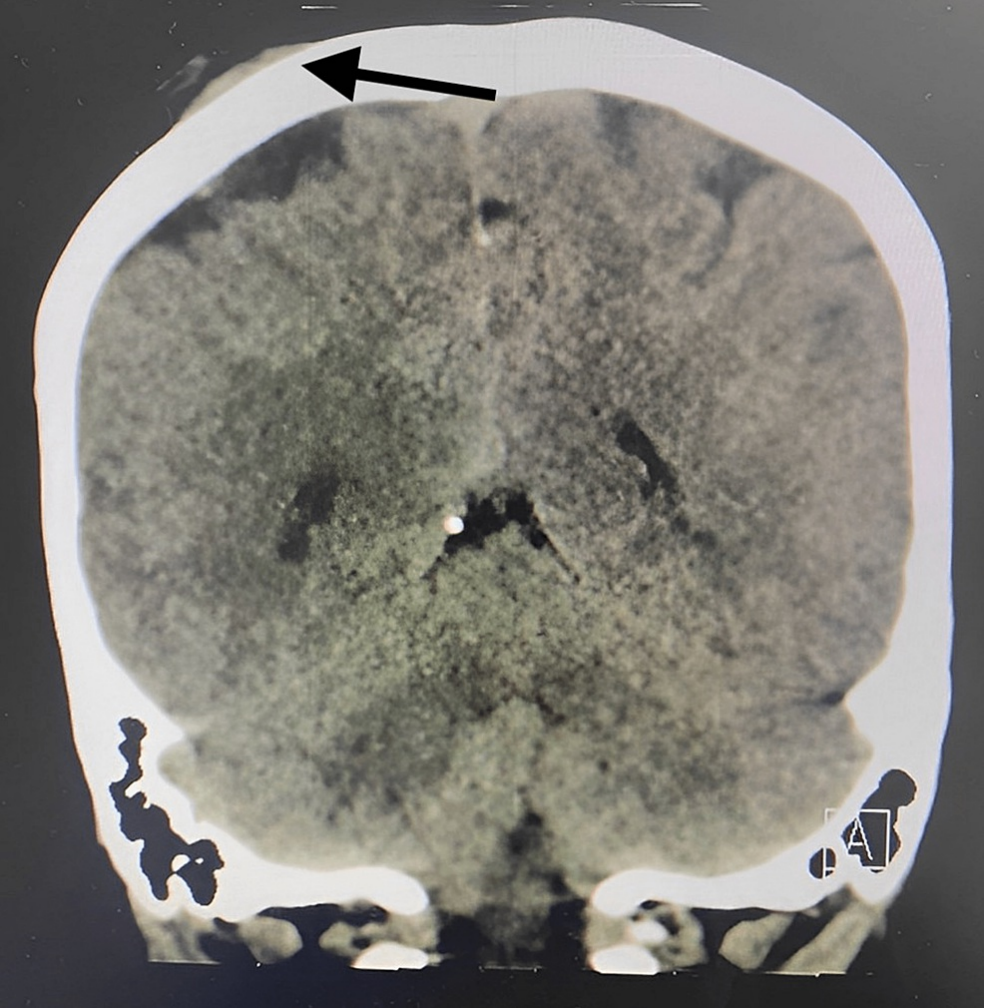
CT scan of the brain without contrast (coronal view) showing right scalp swelling without bone destruction or intra-cranial extension
